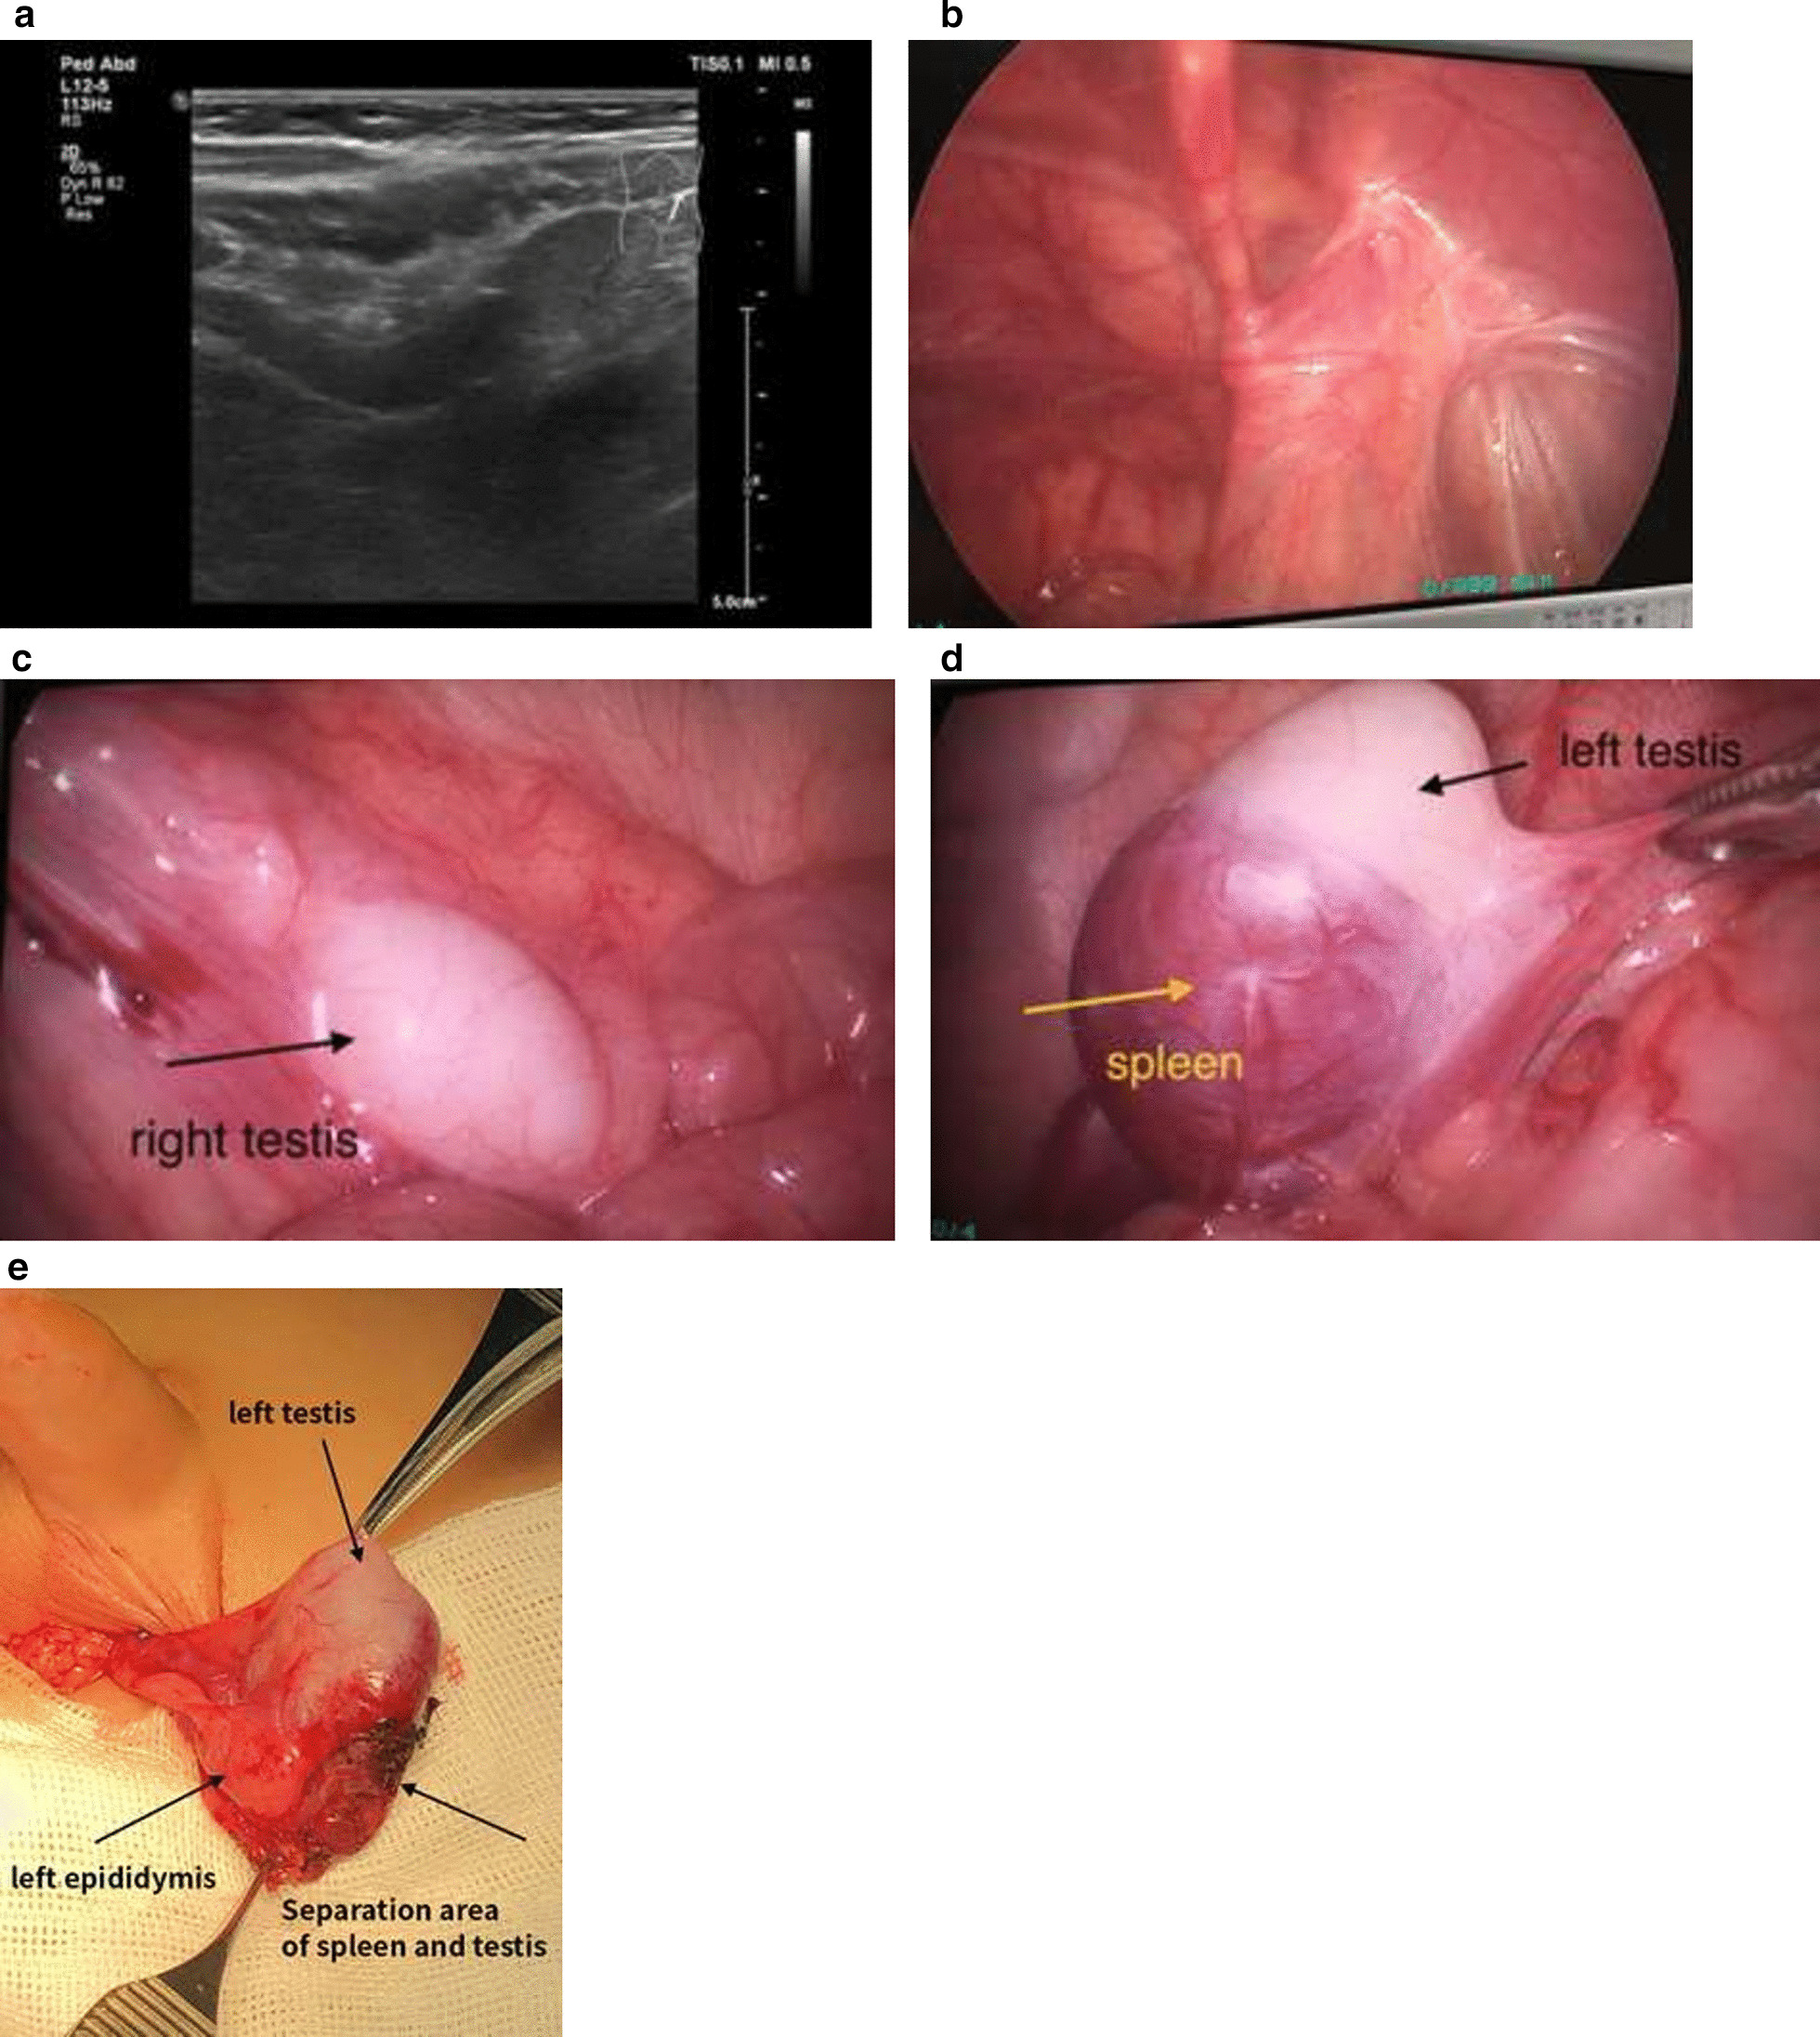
Continuous splenogonadal fusion on the left side and undescended right testis in an 8-month old male infant. a Pre-operative B-mode ultrasound revealed possible fusion of the left testis and the kidney; b single site laparoscopic examination revealed closed right internal ring and the right testis in the iliac fossa, at about the same height as the junction between the iliac vessel and the right vas deferens; c single-site laparoscopic examination visualized the right testis located in the right iliac fossa, approximately 2 cm above the right internal ring, with poorly developed right spermatic vessel; d complete fusion of the spleen and the left testis in the left iliac fossa visualized by the single-site laparoscopic examination; e the left testis descended into the scrotum after being separated from the spleen
